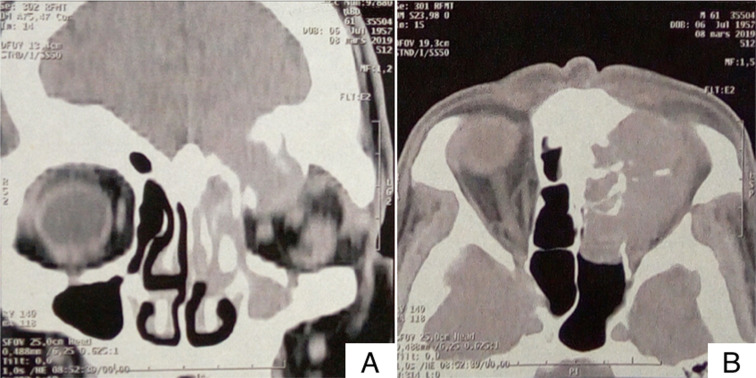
A) axial; B) coronal view of computed tomography showing voluminous spontaneously hyperdense mass taking weak contrast, occupying the ethmoid, maxillary and frontal sinus with bony destruction of the left ethmoidal blade and the roof of the left orbit
